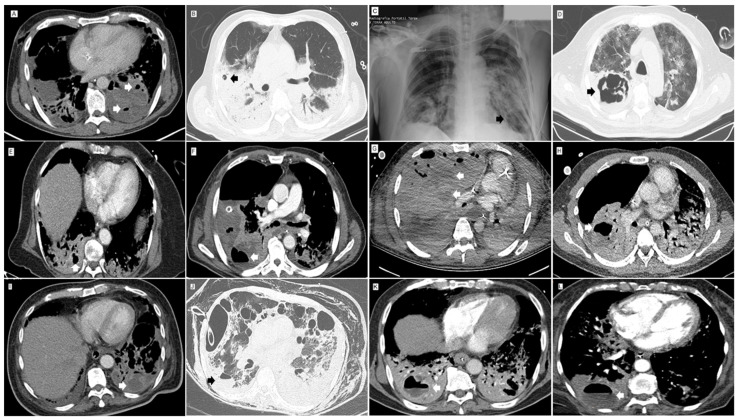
Lung images of post-COVID-19 necrotizing pneumonia. (A) Contrast CT. Mediastinal Window. Low density area of the left lower lobe lung parenchyma with loss of parenchymal enhancement, with architectural distortion and small abnormal gas-filled spaces (arrows) due to necrotizing pneumonia. (B) Non-contrast CT. Lung Window. Small round 9 mm thin-walled, air-filled lesion (pneumatocele) (arrow) in a right upper lobe area of consolidation. (C) Chest X-Ray. Well-defined 20 mm thin-walled area of parenchymal lucency (arrow) within area of consolidation in the left lower lobe. Pneumothorax. (D) Non-contrast CT. Lung Window. Thick-walled and irregular cavity (arrow) with septum due to lung abscess within area of necrotizing pneumonia. (E) Contrast CT. Mediastinal Window. Heterogeneous attenuation of the right lower lobe lung parenchyma with a consolidation pattern with low-density areas, loss of parenchymal enhancement and small air-filled rounded lesions (arrow) due to necrotizing pneumonia. (F) Contrast CT. Mediastinal Window. Large low-density area with loss of parenchymal enhancement of the right upper lobe lung parenchyma associated with thin-walled air-fluid level cavity due to necrotizing pneumonia with lung abscess. Large air-fluid level (arrow) in the pleural space due to empyema. (G) Contrast CT. Mediastinal Window. Large area with increase in parenchymal attenuation with a consolidation pattern and low-density areas (arrows), loss of parenchymal enhancement, architectural distortion, and peripheral air-filled rounded lesions due to necrotizing pneumonia. (H) Contrast CT. Mediastinal Window. Low-density area with loss of parenchymal enhancement in the right lower lobe, associated with adjacent cavitary lesion (arrow) due to necrotizing pneumonia and lung abscess. (I) Contrast CT. Mediastinal Window. Area of consolidation in the left lower lobe with low-density areas (arrow) of liquid content with subtle peripheral enhancement due to necrotizing pneumonia with abscess. (J) Non-contrast CT. Lung Window. A 3-cm thin-walled collection with air-fluid level (arrow) due to superinfection of a lung cavity or abscess in the right lower lobe. (K) CT pulmonary angiography. Mediastinal window. Bilateral lower lobe consolidation and collection with air-fluid level (arrow) with peripheral enhancement in right lower lobe due to lung abscess. (L) CT pulmonary angiography. Mediastinal window. Collection with air-fluid level (arrow) with low peripheral enhancement in right lower lobe consolidation due to lung abscess.
